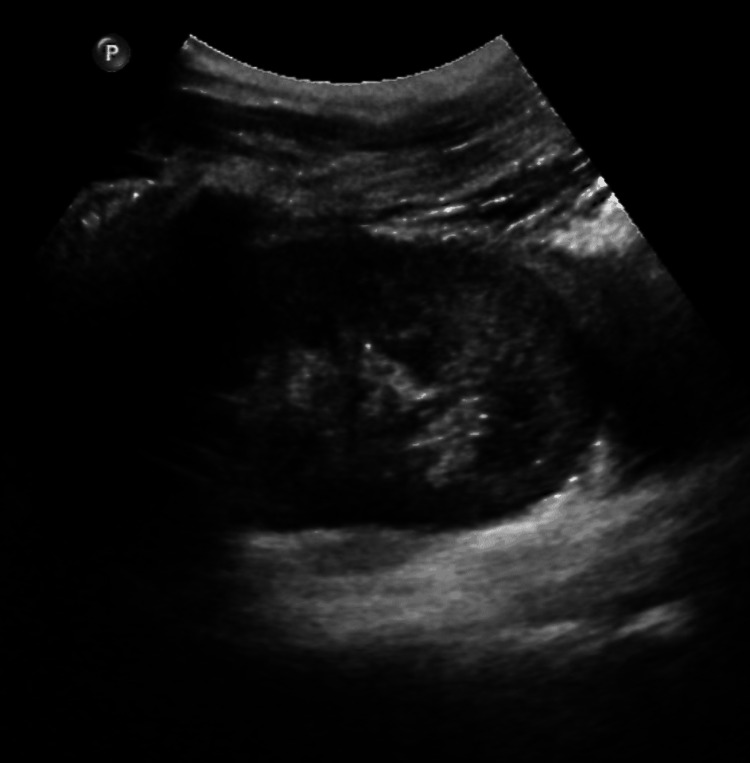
Ultrasonogram of the right kidney post therapy showing resolved hydronephrosis
